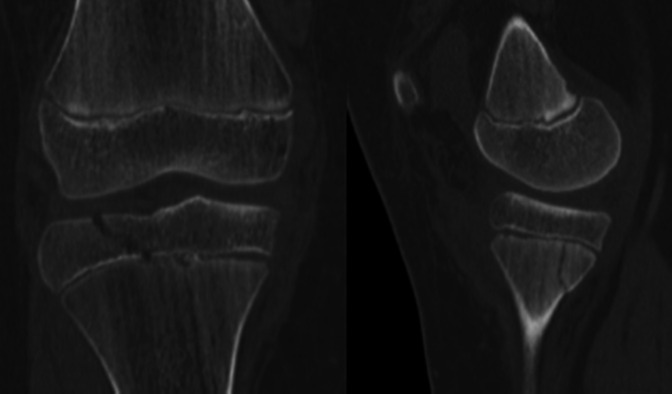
CT scan further defining the injury.
